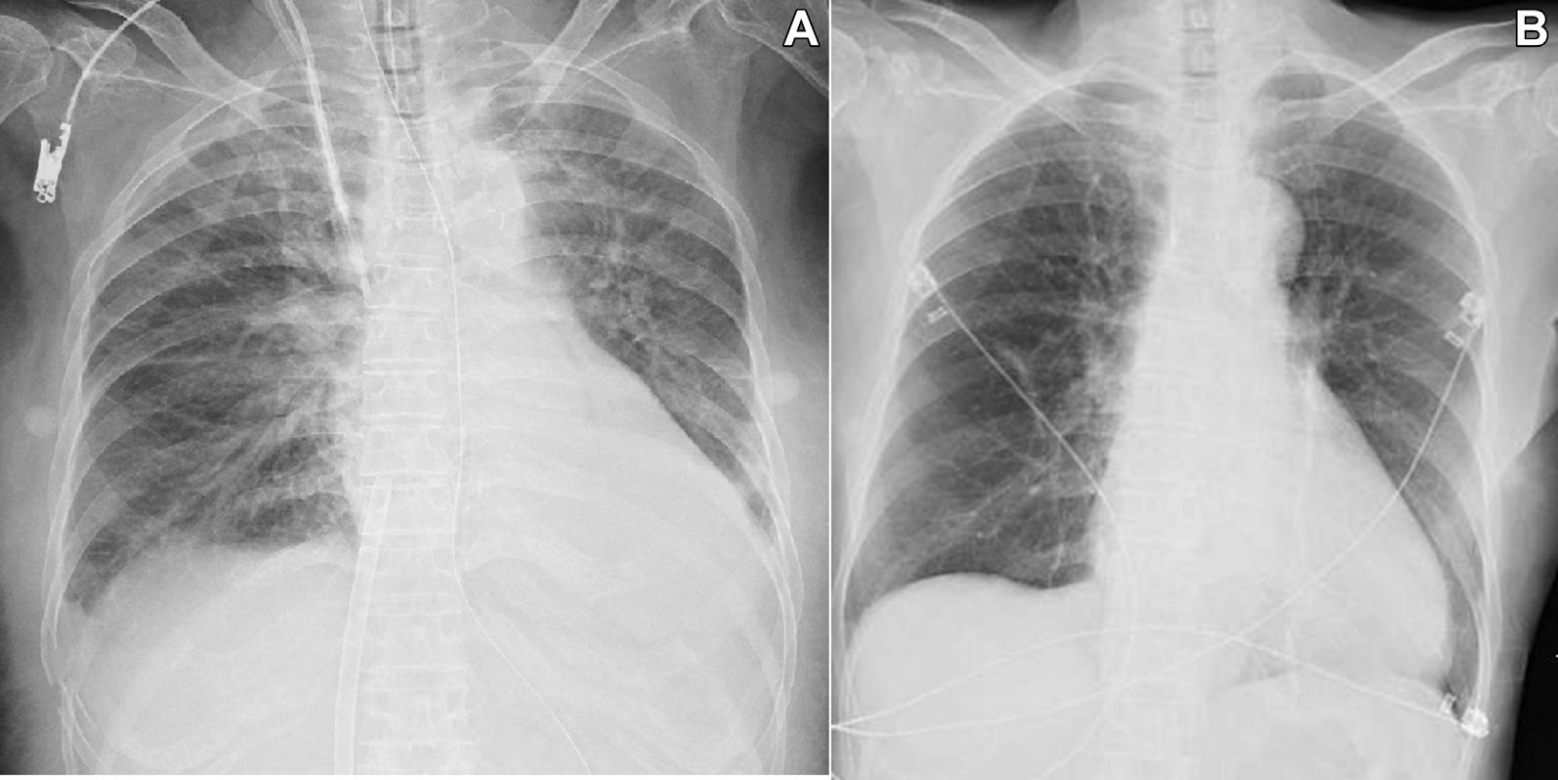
Chest X-Ray Films Before and After Percutaneous Transluminal Septal Myocardial Ablation (A) Chest x-ray film showing bilateral pulmonary congestion and pleural effusion. The cardiothoracic ratio is approximately 60%. (B) Bilateral pulmonary congestion and pleural effusion are no longer observed after percutaneous septal myocardial ablation.
